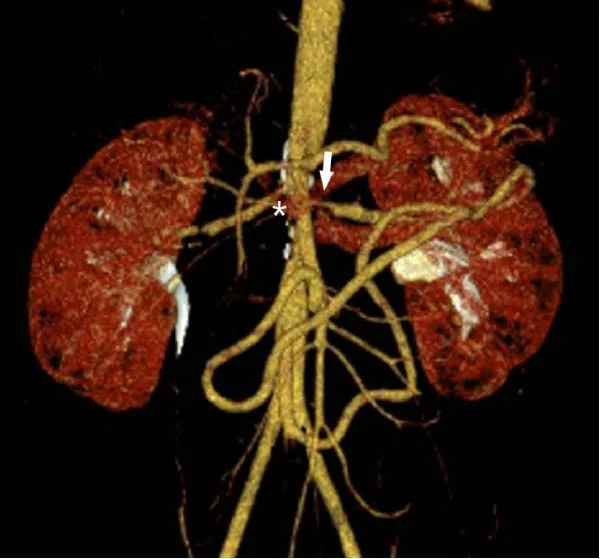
CT angiography, 3D‐reconstruction, ap view—both renal arteries showing signs of stenosis, left (arrow) is more pronounced than right (asterisk).
